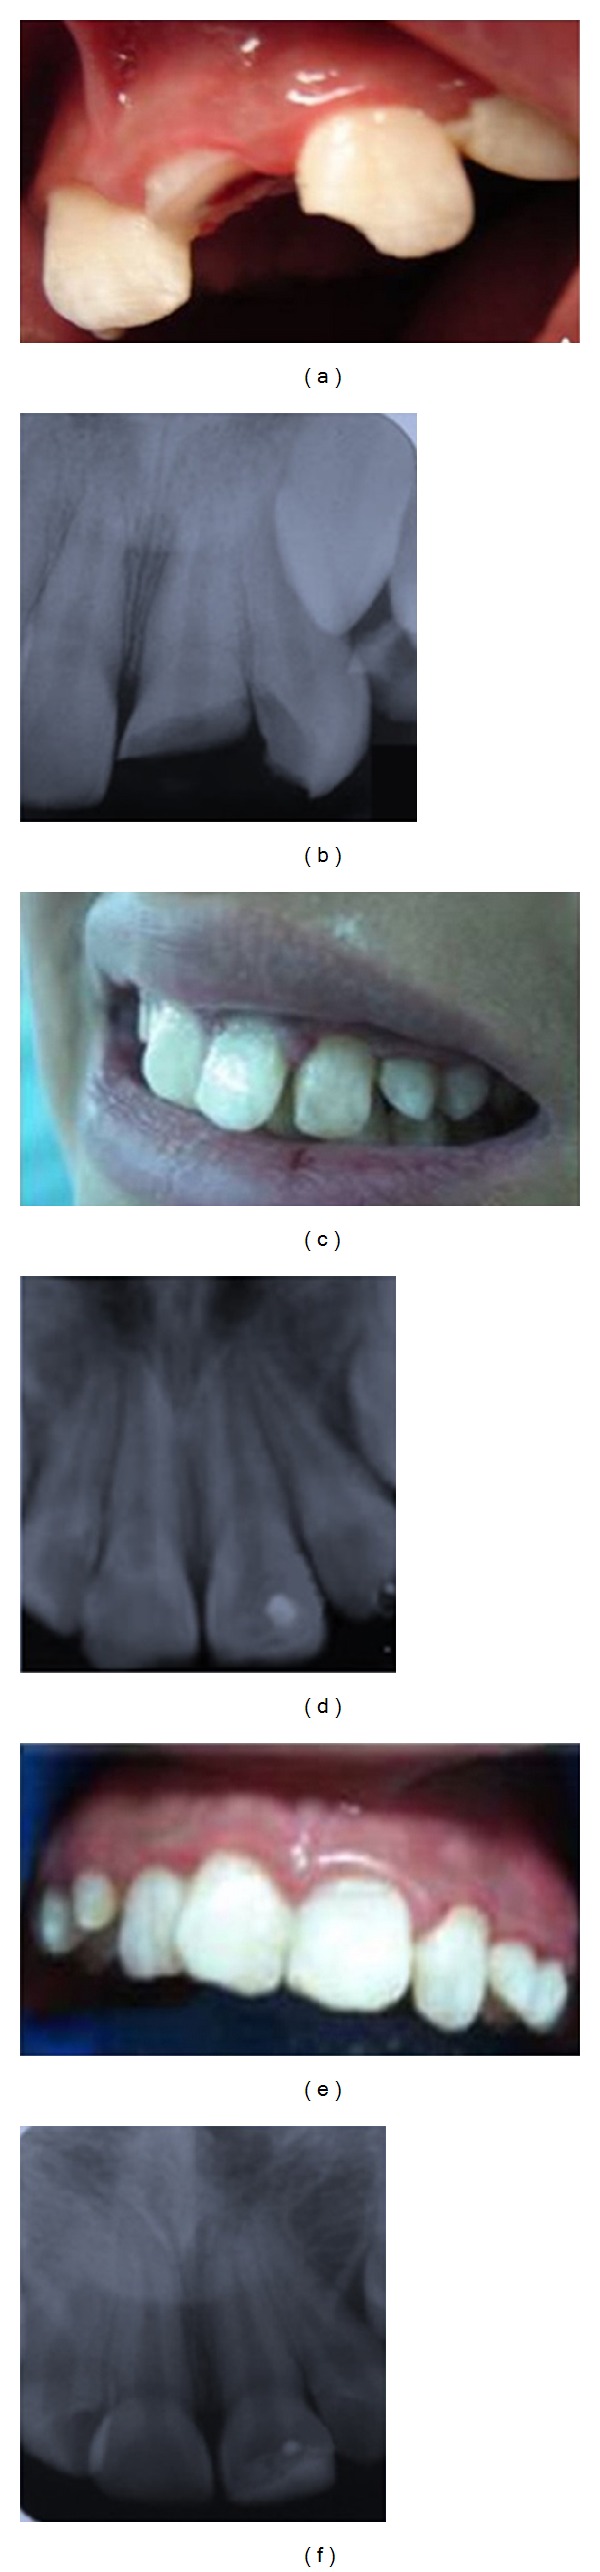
(a) Initial clinical image of patient 4 h after trauma with complicated crown fracture in maxillary left central incisor, with ulcerated and exposed pulp, and enamel crown fracture in left lateral incisor. (b) Initial radiograph showing loss of dental structure, complete root development, closed apices, normal periodontal ligament, and absence of root or alveolar bone fractures. (c) Resin reconstruction and reattachment of tooth fragments after treatment. (d) Posttreatment radiograph showing indirect pulp capping with resin reconstruction in the maxillary left lateral incisor and pulpotomy with reattachment of the dental fragments in the left central incisor. (e-f) Clinical and radiographic examinations 6 months after trauma. Patient showed no periodontal or periapical pathology, nor pulpal signs or symptoms.
